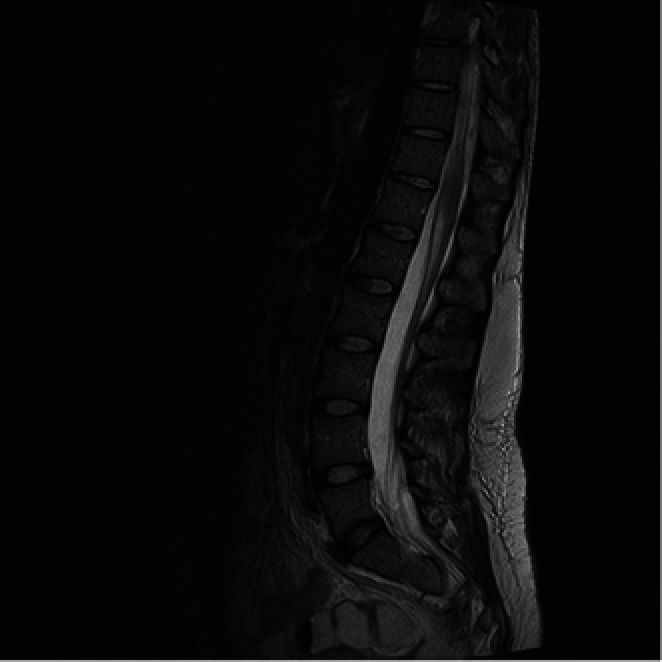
FIGURE 3 Conus medullaris involvement in acute inflammatory myelopathy. Sagittal T2‐weighted lumbar spine magnetic resonance imaging demonstrates hyperintense signal changes and mild enlargement of the conus medullaris, with associated thickening of the cauda equi.
